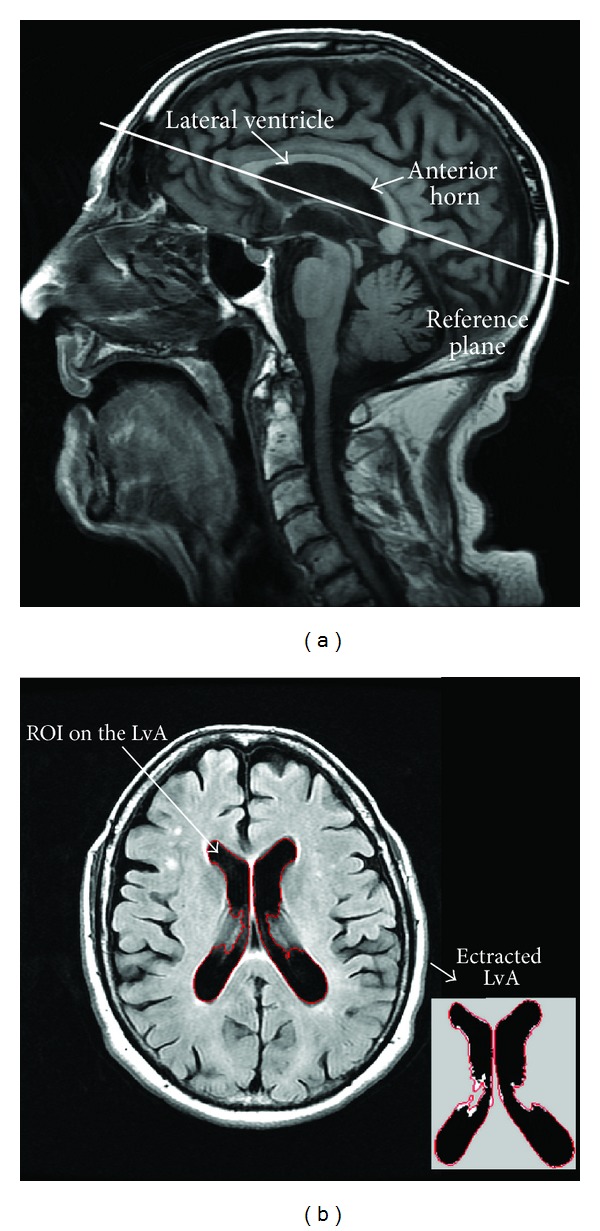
(a) Sagittal T1-weighted image showing the reference plane intersecting the anterior horn and the body of the lateral ventricles. (b) Segmentation of the lateral ventricles on the corresponding 2D FLAIR slice using the level set algorithm then thresholding to draw a region of interest and extract the lateral ventricles area.
